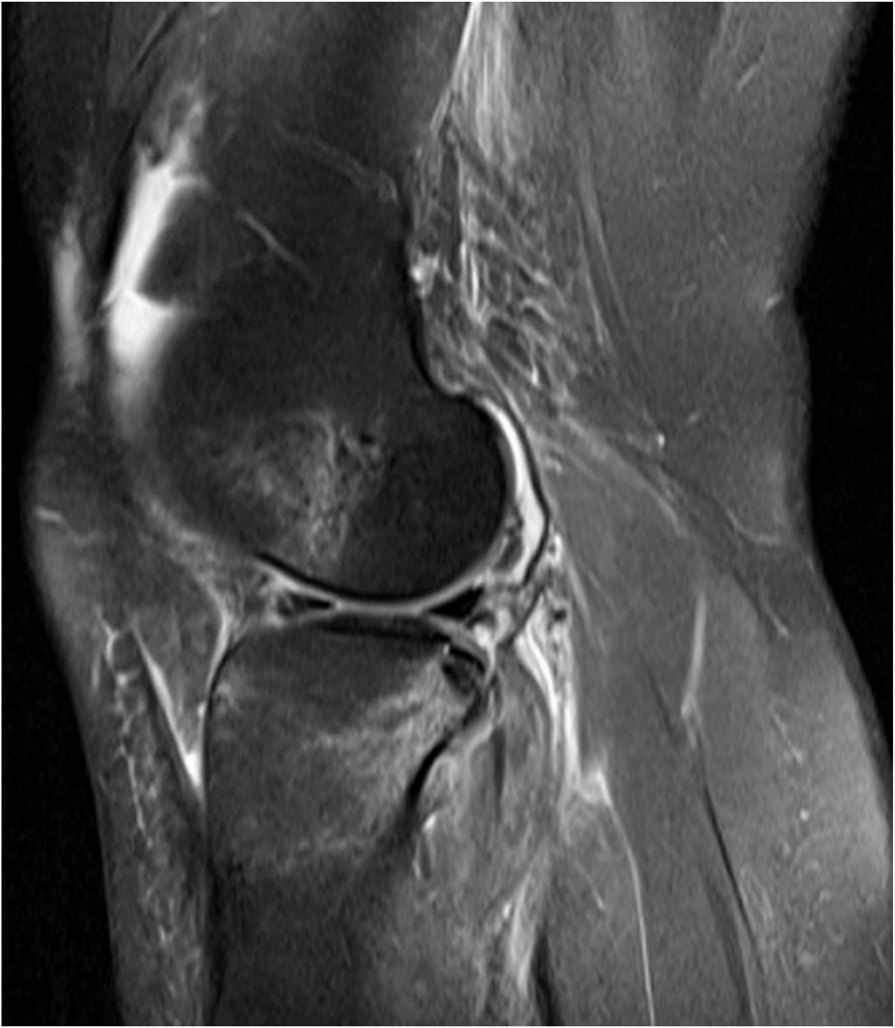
MRI post-ACL rupture demonstrating severe bruising in the lateral femoral condyle and posterior tibial plateau
